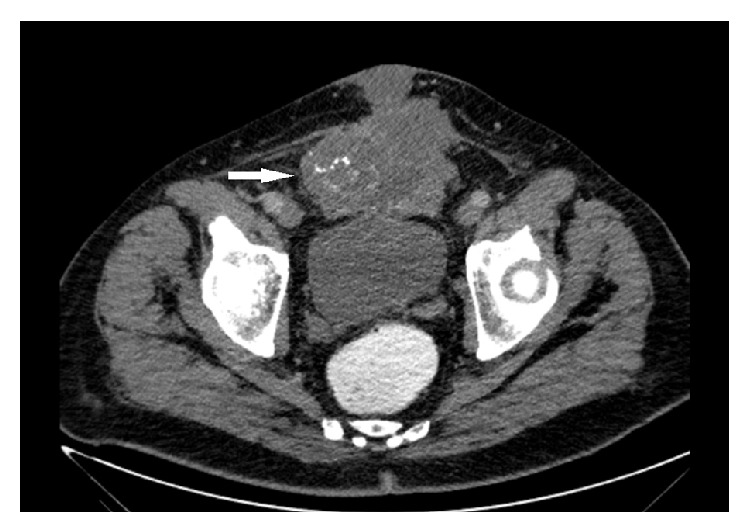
Cross-section contrast-enhanced computed tomography (CECT). There is a heterogeneous lesion (arrow) filling the bladder, extending to the anterior, and containing punctate amorphous calcifications.
